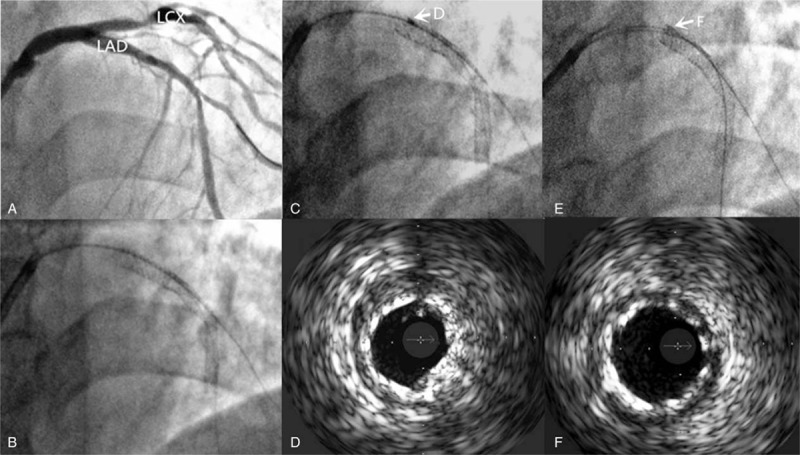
Percutaneous intervention of left anterior descending (LAD) artery. (A) 70%, type A lesion of medial LAD. (B) Two stents placement. (C) Longitudinal compression of the proximal stent as marked by a white arrow. (D) Intravenous ultrasound was performed immediately after longitudinal compression. (E) Post stent deployment, the longitudinally compressed proximal part of stent as marked by a white arrow. (F) Intravenous ultrasound was performed after dilation.
